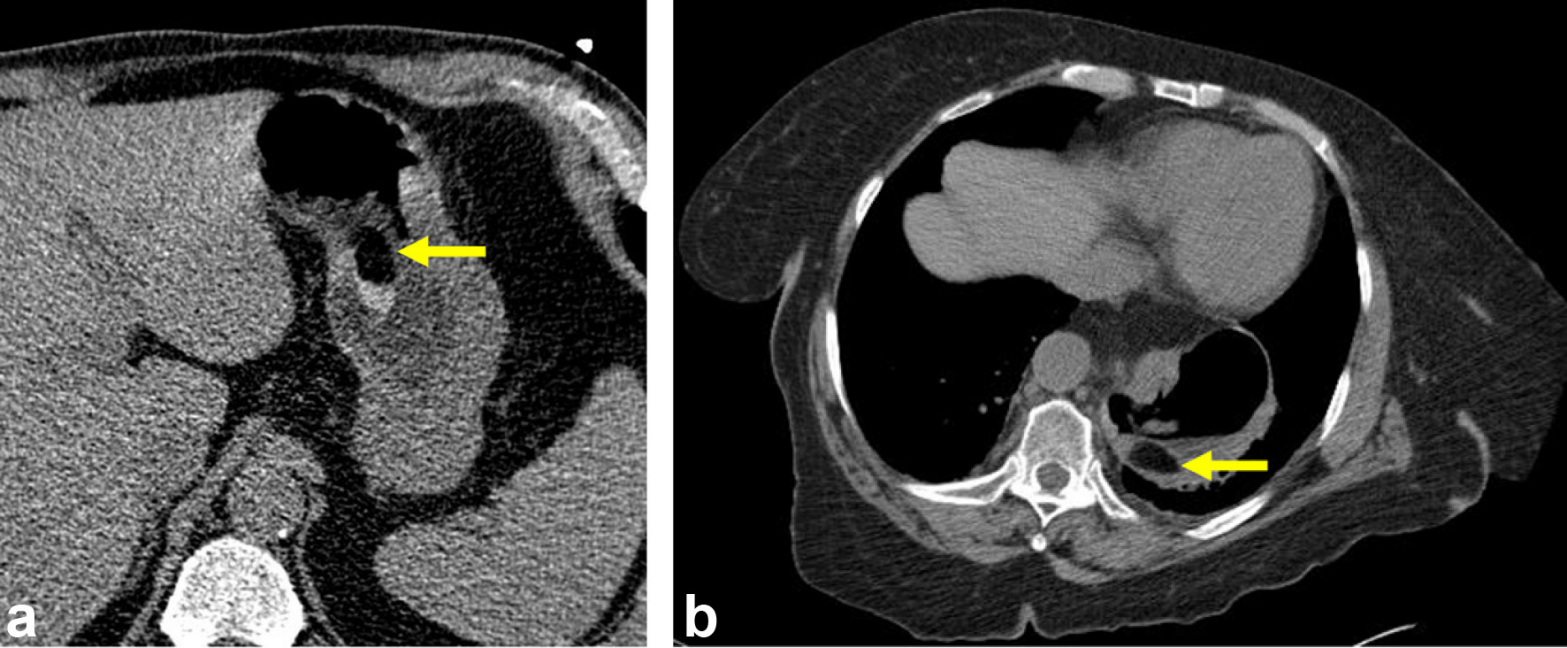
Diagnosis of gastric lipoma by low-dose protocol CT (Figure 2 for low-dose imaging from a PET-CT) (a) Axial imaging from a coronary
